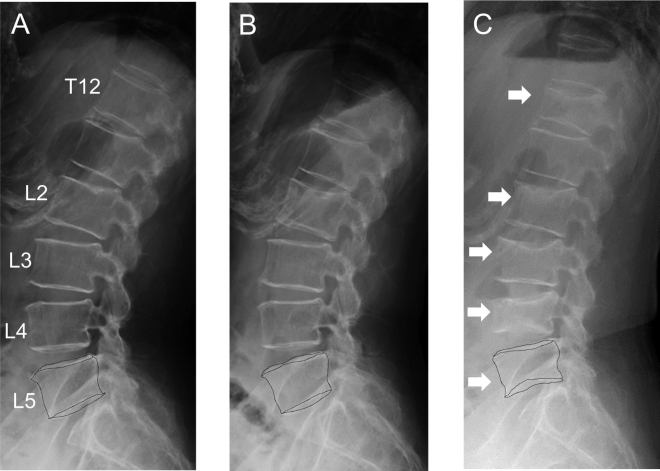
Lateral spine radiographs with the patient standing. (A) Before romosozumab treatment, (B) 2 months after romosozumab treatment, (C) 4 months after romosozumab treatment. Time-course radiographs revealed five new vertebral fractures with vertebral deformity (T12, L2, L3, L4, and L5). Dot lines show the outline of L5 vertebral body.
